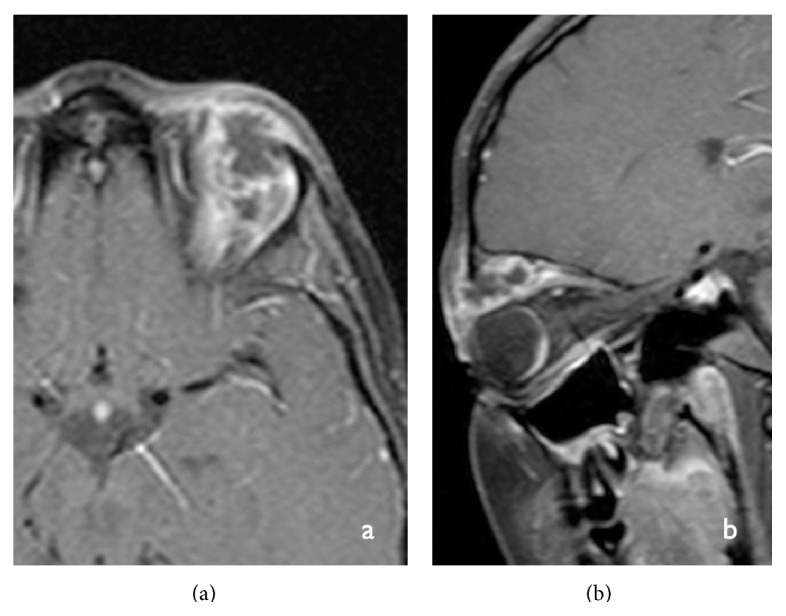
Contrast-enhanced axial (a) and sagittal T1-weighted MRI image showing the choroidal retinal mass and the peripherally enhancing lobulated lesion in the superior lateral extraconal plane centered in the region of the left lacrimal gland.
